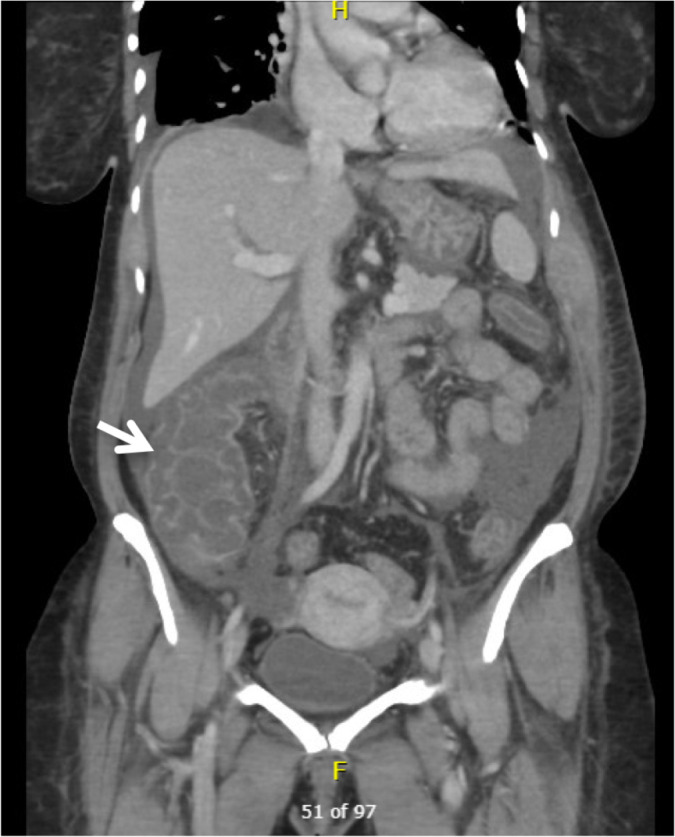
CT Angiogram of coronal view. The abdomen shows mural extensive thickening associated with submucosal oedema of colon (arrow).
